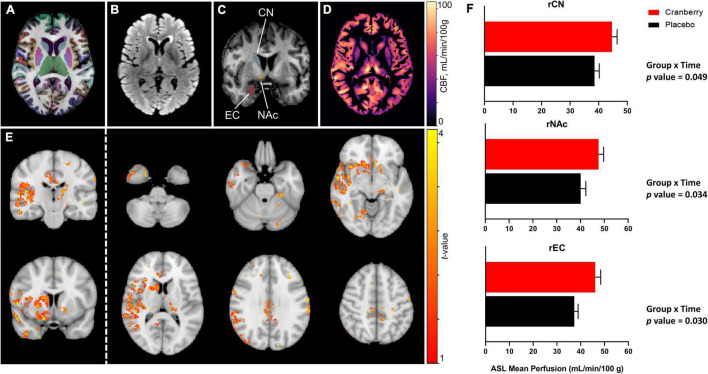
Representative magnetic resonance imaging data following 12-week consumption of a cranberry extract or a placebo. (A) Axial view of FastSurfer cortical and subcortical segmentations superimposed on a T1-weighted image; (B) axial T2-weighted fluid attenuated inversion recovery (FLAIR) image; (C) a coronal view of the T1-weighted image indicating regions that showed significantly increased perfusion after 12 weeks consumption of a cranberry extract—namely, from superior to inferior, the right caudate nucleus, accumbens area, and entorhinal cortex; (D) an axial cerebral blood flow (CBF) map, in the T1 space, derived from arterial spin labelling data; (E)
